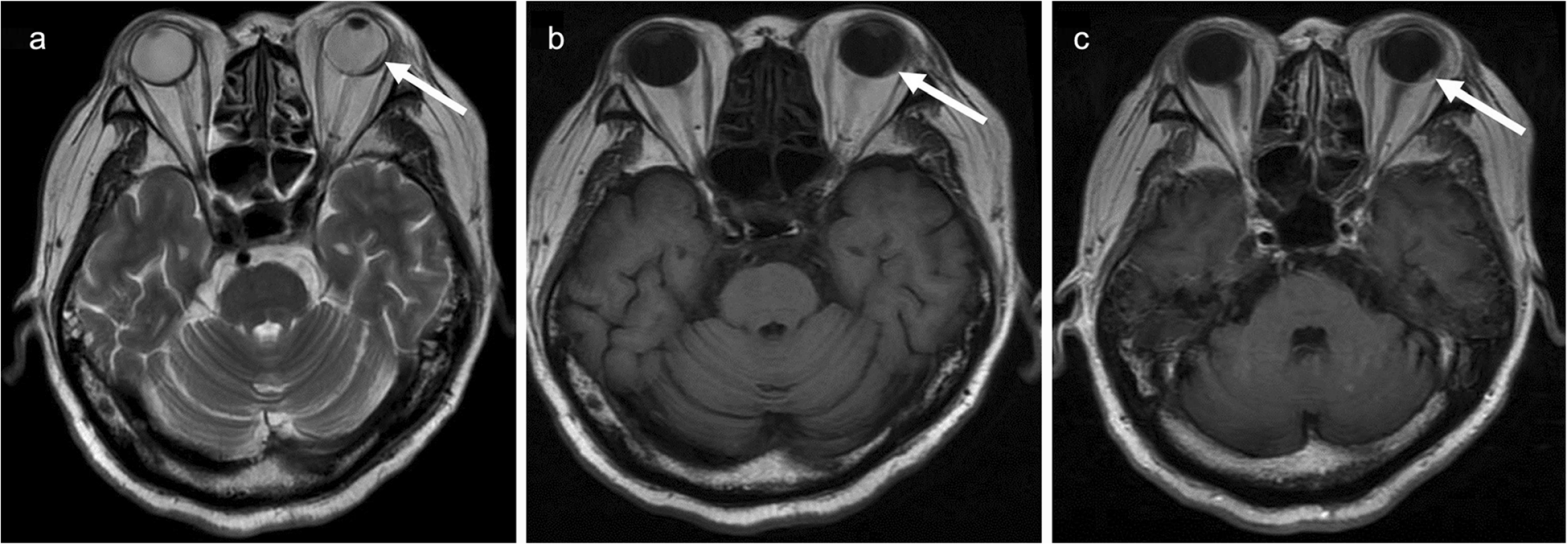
Magnetic resonance imaging of the orbit. An abnormal lesion was noted behind the left eye (shown on arrow) with a high-intensity signal on T2-weighted images (a), low signal intensity on T1-weighted images (b) and high signal intensity on gadolinium contrast T1-weighted images (c)
